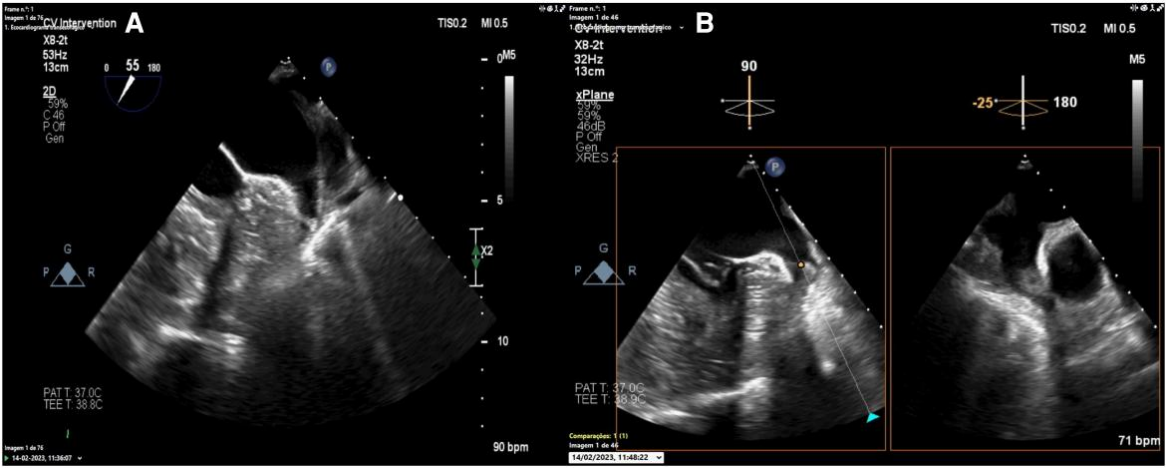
Intraprocedural transoesophageal echocardiography. (*A*) No thrombus in left atrial appendage before femoral puncture. (*B*) Thrombus in formation in the left atrial appendage was detected after femoral puncture demonstrating the high prothrombotic risk of this patient.
